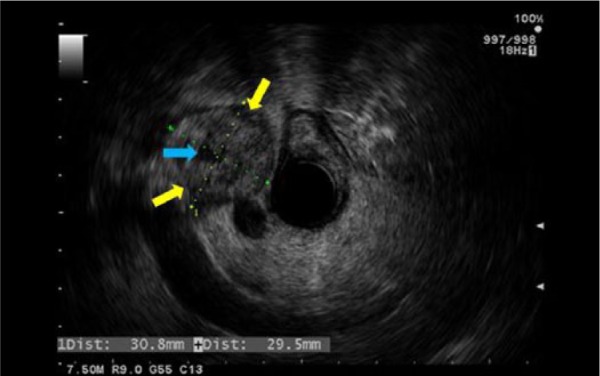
Endosonographic view of the enlarged para-pancreatic lymph node. Description: Endoscopic ultrasound demonstrates a well-defined para-pancreatic head hypoechoic lymph node (LN) measuring 30.8 ′ 29.5 mm (yellow arrow). This LN shows small central anechoic area suggestive of necrosis (blue arrow).
